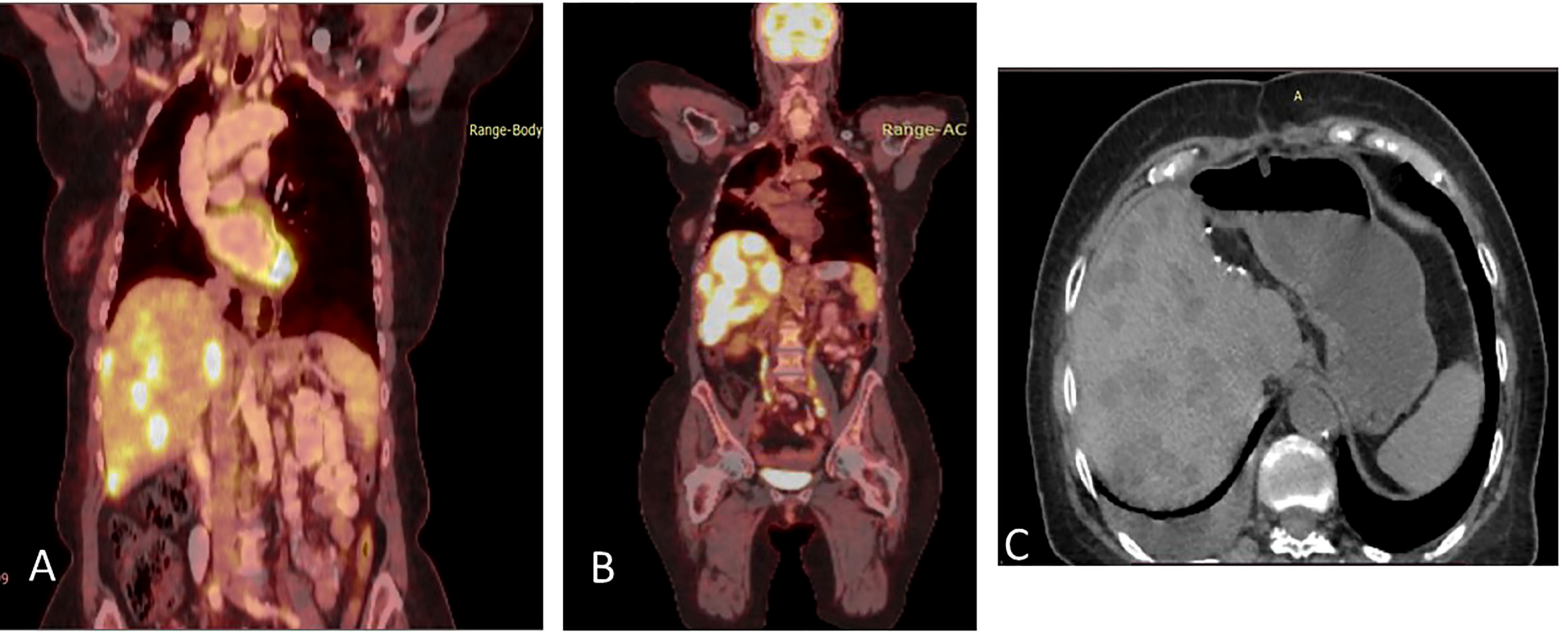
(A) Coronal section, superimposed PET-CT scan, December 2020. Four years after the first presentation of stage 3B NSCLC, the right lung shows a significant reduction in FDG-18 uptake and decreased tumor size, with only a scar, indicating the lung’s complete response to treatment. However, status-post left hepatectomy, there is increased FDG-18 uptake in the right lobe of the liver, consistent with multifocal right hepatic metastatic disease. (B) Two months later, February 2021, there is further progression in size, number, and metabolic activity of metastases throughout the right lobe of the liver, indicating more aggressive disease. There remains no evidence of recurrent or residual disease in the lung. (C) Non-contrast, axial CT scan from April 2021 shows interval regression in size and number of liver masses. The white spots are sutures from the surgery to remove the left hepatic lobe (status-post left hepatectomy).
